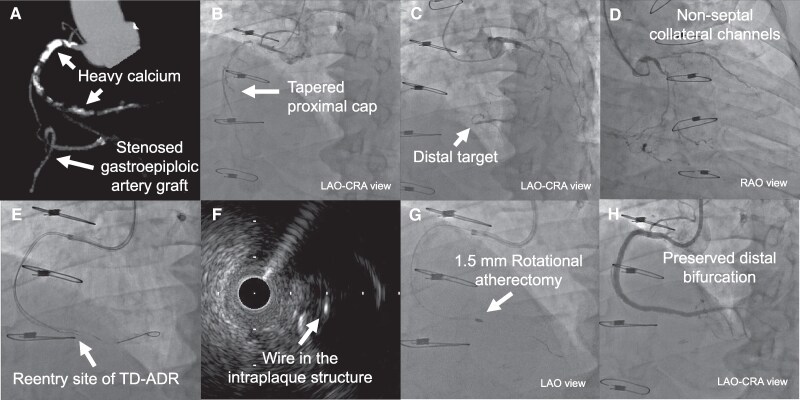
Images of patient 1: (*A*) CT angiogram showing heavy calcification and a stenosed gastroepiploic artery graft. (*B*) Right coronary angiography demonstrating a tapered proximal cap. (*C*) Left coronary angiography highlighting the distal target. (*D*) Left coronary angiography showing non-septal collateral channels. (*E*) Re-entry site of tip detection-antegrade dissection and re-entry (TD-ADR). (*F*) A Conquest Pro 12ST guidewire in the intraplaque structure. (*G*) Rotational atherectomy using a 1.5 mm burr for the distal uncrossable lesion. (*H*) Final angiogram showing a preserved distal bifurcation.
